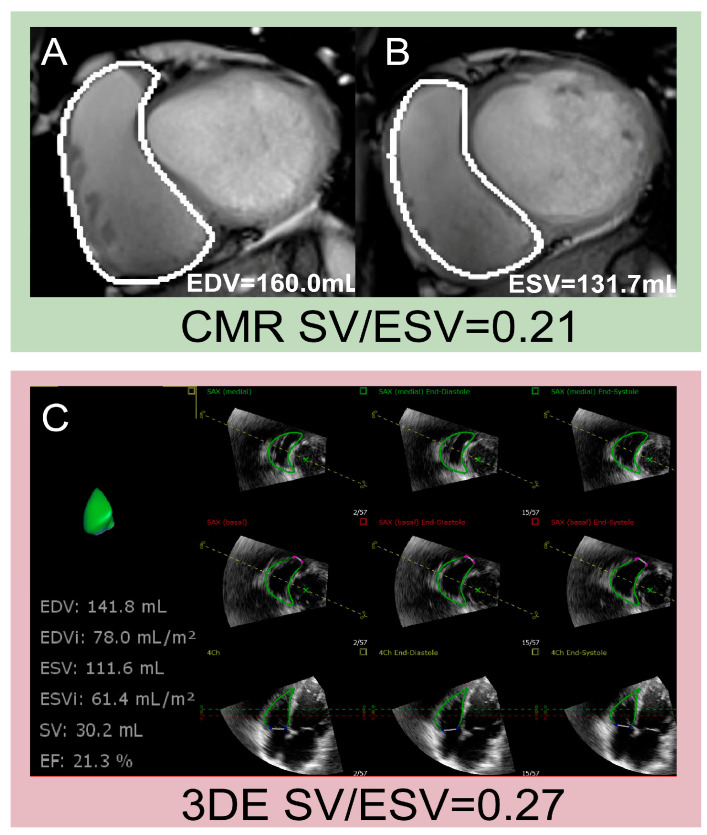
Examples of assessments of SV/ESV using CMR and 3DE. (A,B) Measurement of EDV, ESV, and SV using CMR; (C) measurement of EDV, ESV and SV using 3DE. 3DE: three-dimensional echocardiography; CMR: cardiac magnetic resonance; EDV: end-diastolic volume; ESV: end-systolic volume; SV: stroke volume.
